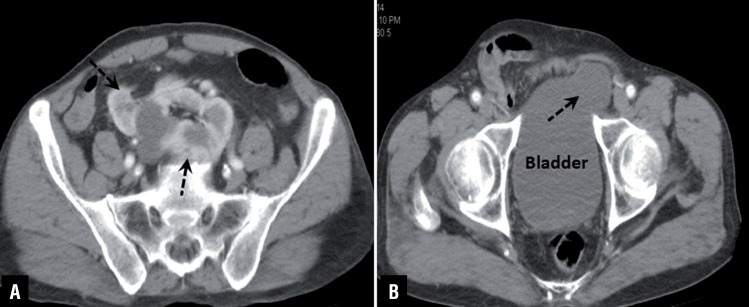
Axial CT images show pancake kidney and bladder herniation (dashed arrows).
